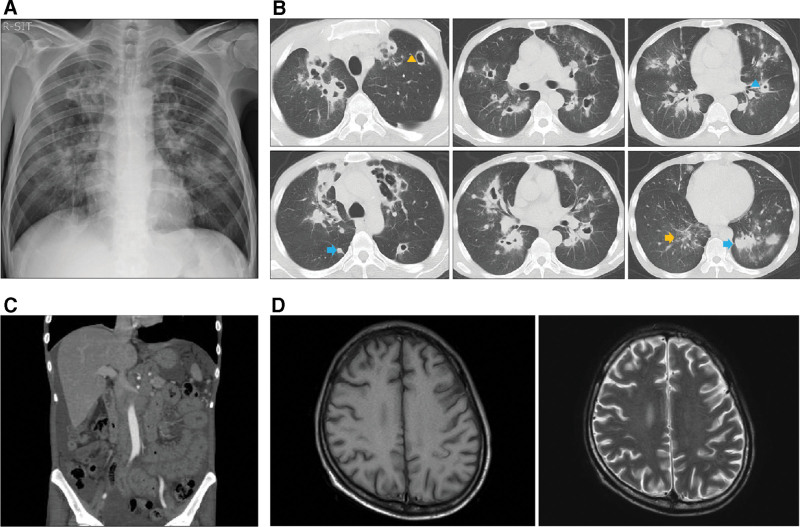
Imaging findings on admission. Chest radiograph revealed cavitary lesions in both lungs (A). Chest computed tomography confirmed multiple cavitary lesions in both lungs, ranging from thin-walled to thick-walled lesions. Nodular (blue arrows) and granular (yellow arrows) opacities with relatively well-defined borders and high contrast were also observed. Many nodules and granules showed a centrilobular distribution, whereas some nodules were suspected to have a random distribution. Cavitary lesions were also observed (yellow arrowhead) (B). Abdominal imaging revealed intra-abdominal lymphadenopathy and edematous thickening of the small intestinal wall (C). White matter lesions were observed in the left parietal lobe (D).
